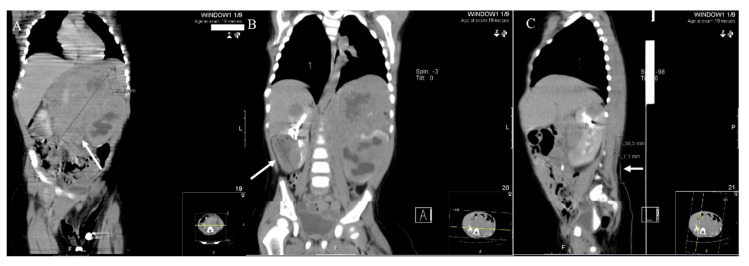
Thoracic, abdominal, and pelvic CT scan performed at diagnosis: (A) large described mass over left kidney (arrow); (B) metastatic lesion on right kidney (arrow); (C) hemorrhagic infiltration of the paravertebral muscles (arrowhead).
